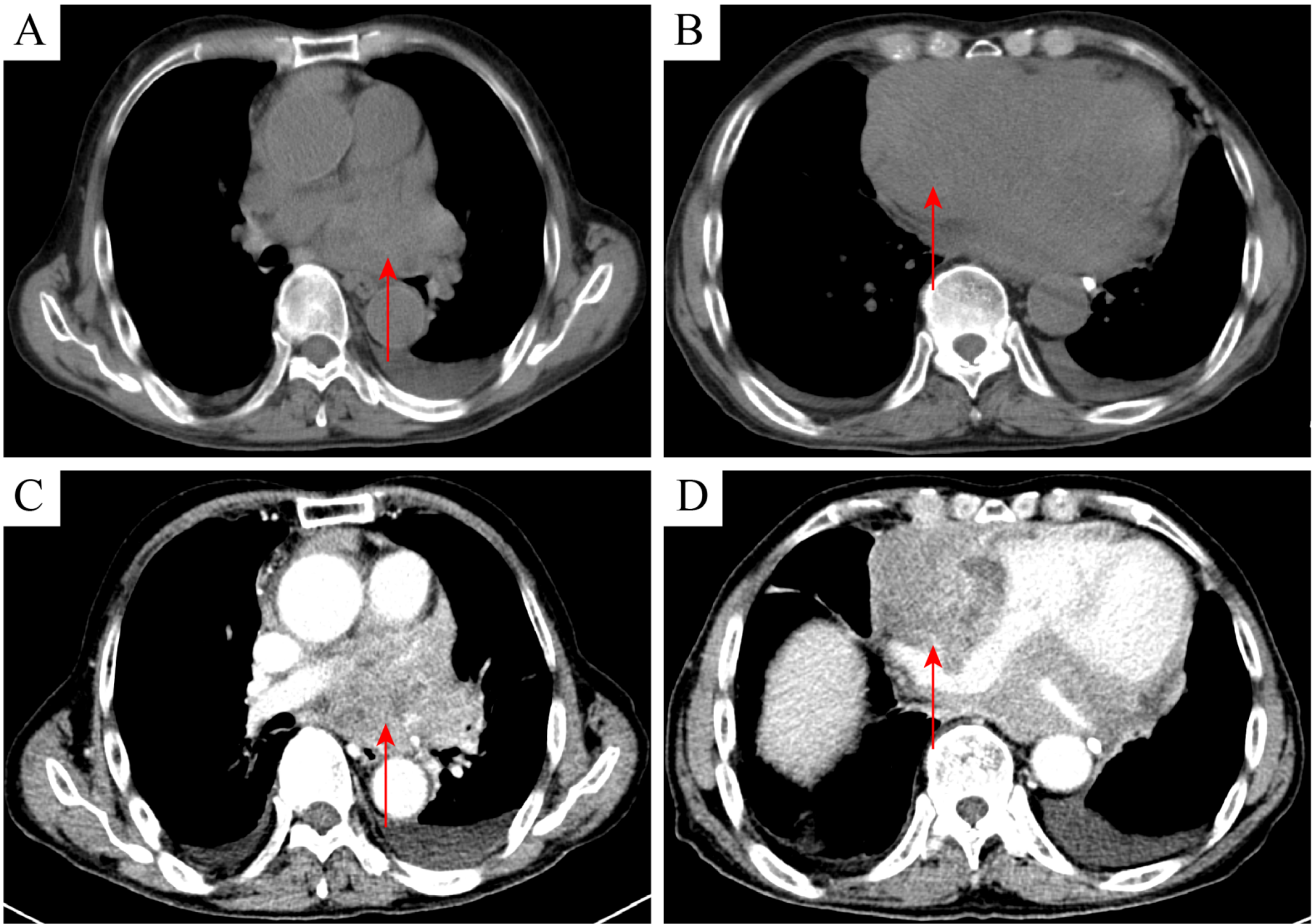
Chest CT plain scan + enhancement demonstrating mediastinal lesions. (A, B) Plain scan of chest CT showed an irregular soft tissue mass in the right anterior mediastinum, middle mediastinum, and posterior mediastinum (arrow), with uneven density and unclear demarcation from the normal mediastinal structure. (C, D) Chest CT enhancement showed uneven enhancement of the mass, narrowed compression of adjacent blood vessels and cardiac cavity, and undersmooth margins.
